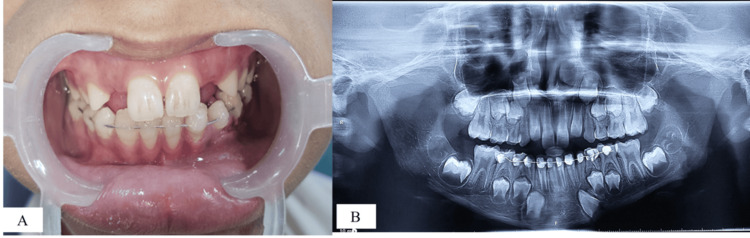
Stabilization using flexible splinting: (A) immediate postoperative clinical photograph; (B) immediate postoperative OPG after flexible splinting OPG: orthopantomogram
